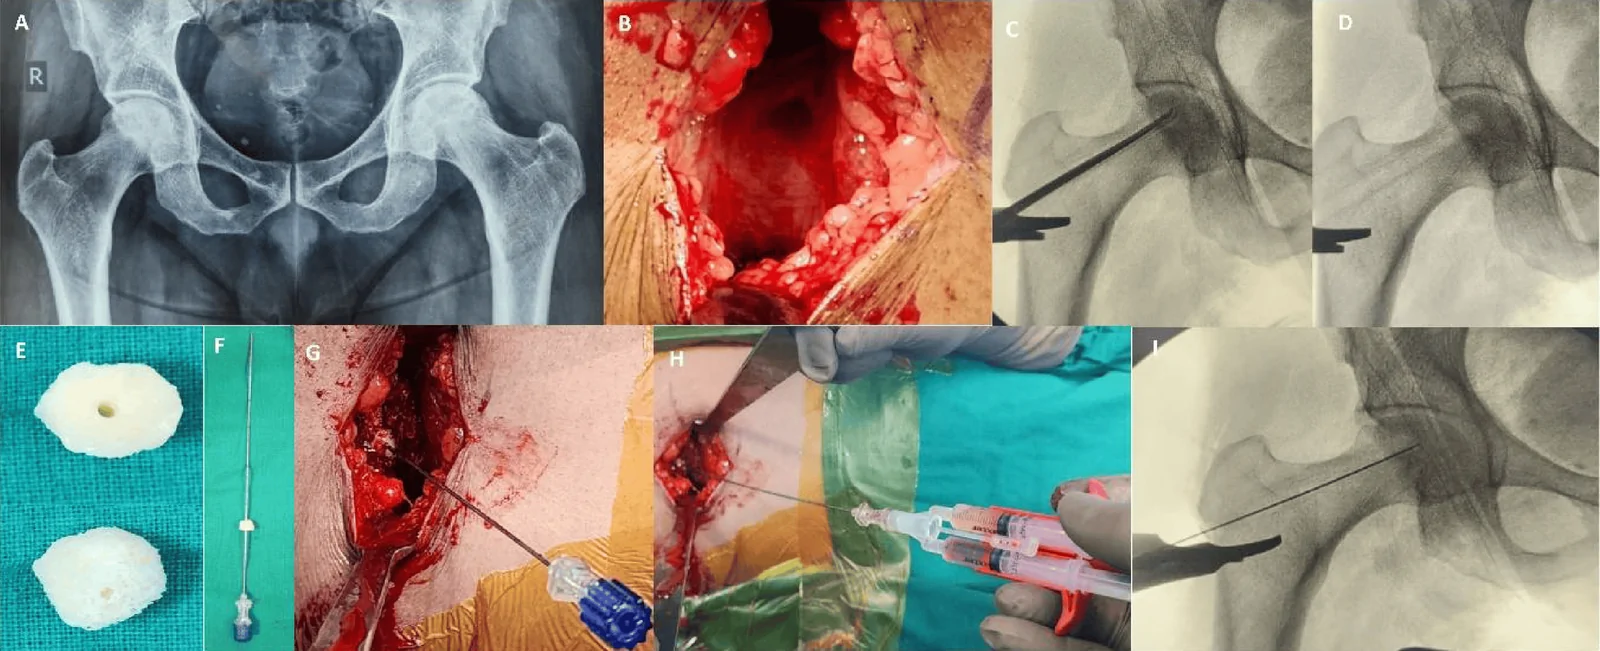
Pre- and intraoperative images of osteoblast cell therapy (A) Preoperative X-ray of a 47-year-old male patient with ONFH; (B) Incision and opening for passing the guide wire; (C, D) Core decompression, a standard procedure, was performed under C-arm guidance, and angular curettes were used to remove sclerotic necrotic bone, creating space for new bone formation; (E) Allograft plug; (F) Allograft plug placed onto the long spinal needle (CE-marked; 18G/20 cm); (G) Long spinal needle positioned for final product implantation; (H, I) Final osteoblast cell–gel mixture delivered to the necrotic site for bone regeneration CE: Conformité Européenne (indicating compliance with European requirements that ensure safety, health, and environmental protection standards); ONFH: osteonecrosis of the femoral head
